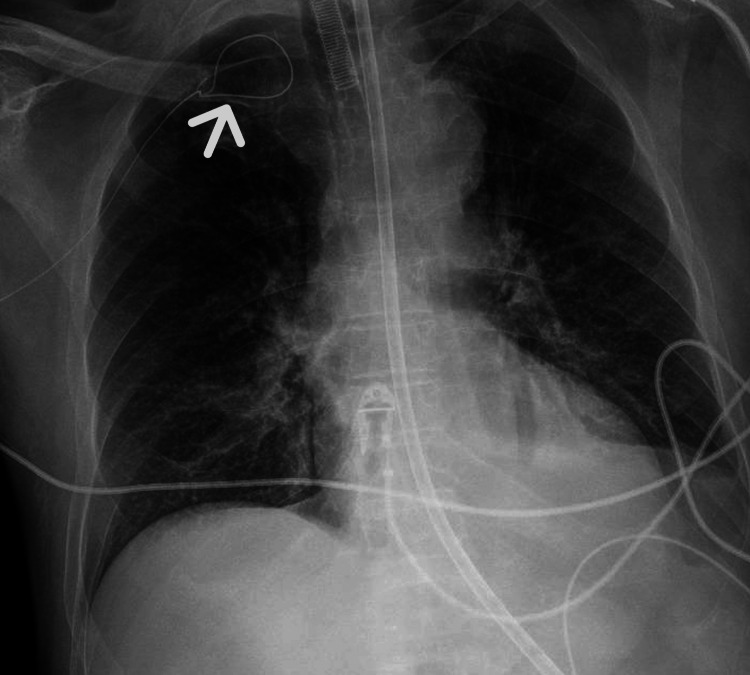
Chest X-ray Chest X-ray showing a looped configuration of the guidewire in the right subclavian region. The arrow indicates the abnormal loop formation beneath the clavicle, suggesting guidewire malposition and prompting further radiological evaluation.
